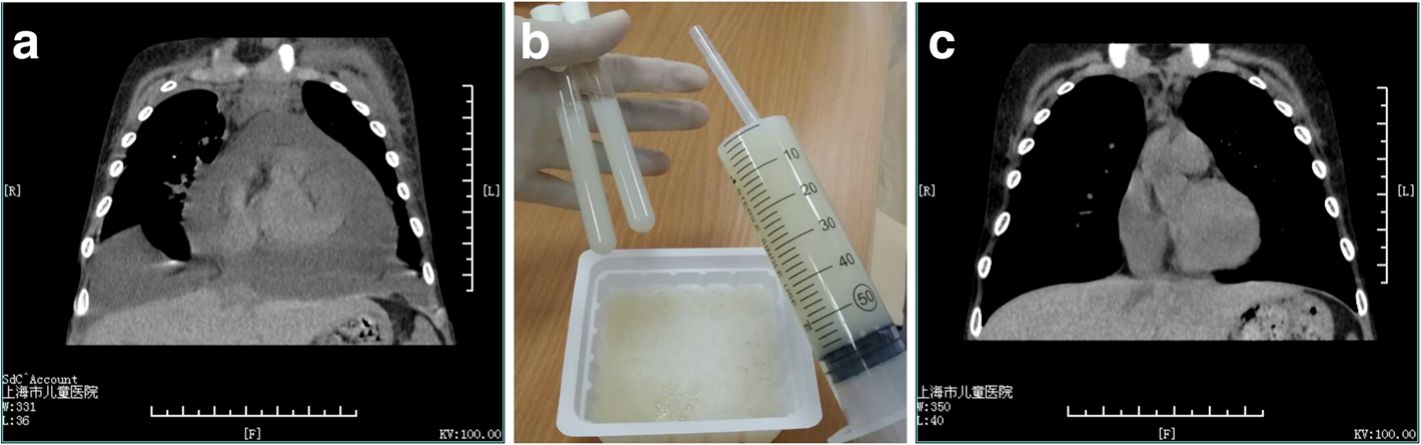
a Thoracic CT demonstrating massive pericardial and pleural effusion. b The milky pericardial effusion was obtained from the child with IgA nephropathy. Laboratory tests revealed that this milky pericardial fluid was saturated chylous effusion. c The thoracic CT demonstrating resolution of pericardial effusion after pericardial drainage and modified diet therapy
